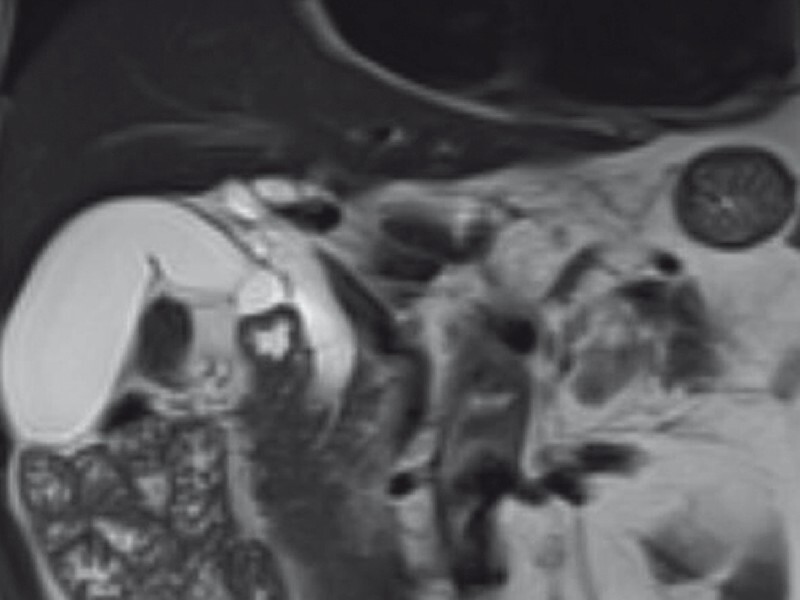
Magnetic resonance imaging revealed a low signal in T2 W with dilation of common bile duct to 12 mm and enlargement of gallbladder.
